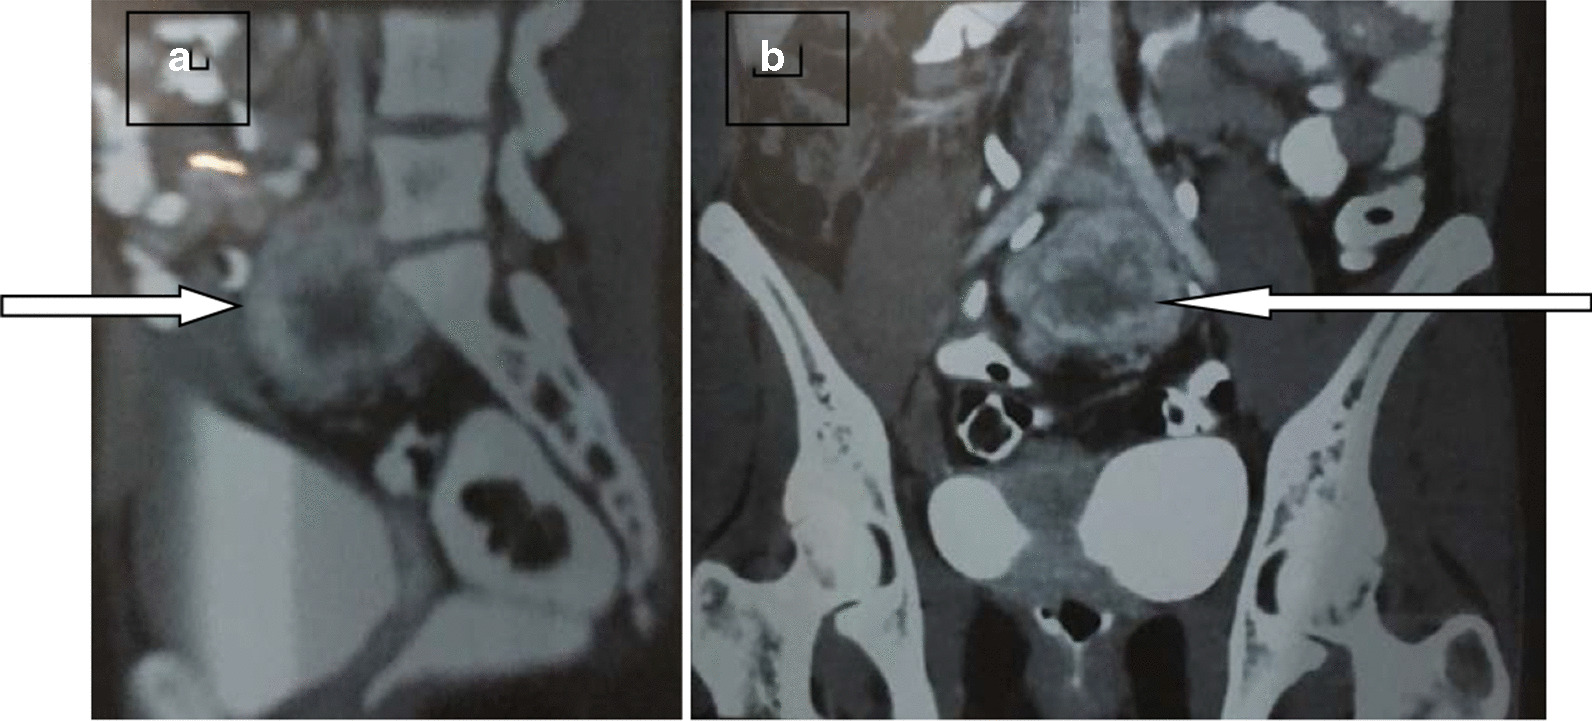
Computed tomography of pelvis with sagittal (A) and coronal sections (B) arrows showing well-defined soft tissue density measuring 5.1 × 5.2 × 4.7 cm with central necrosis
